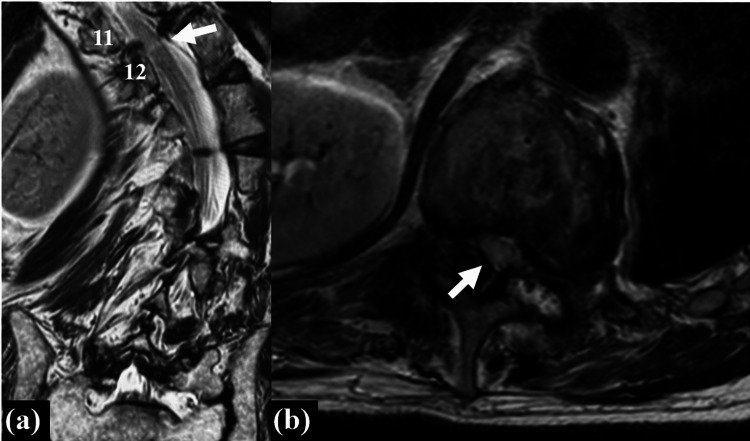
Preoperative T2-weighted magnetic resonance imaging (MRI) (a) (b) T2-weighted MRI showing stenosis with high intramedullary signal at T11–12 (white arrows).
